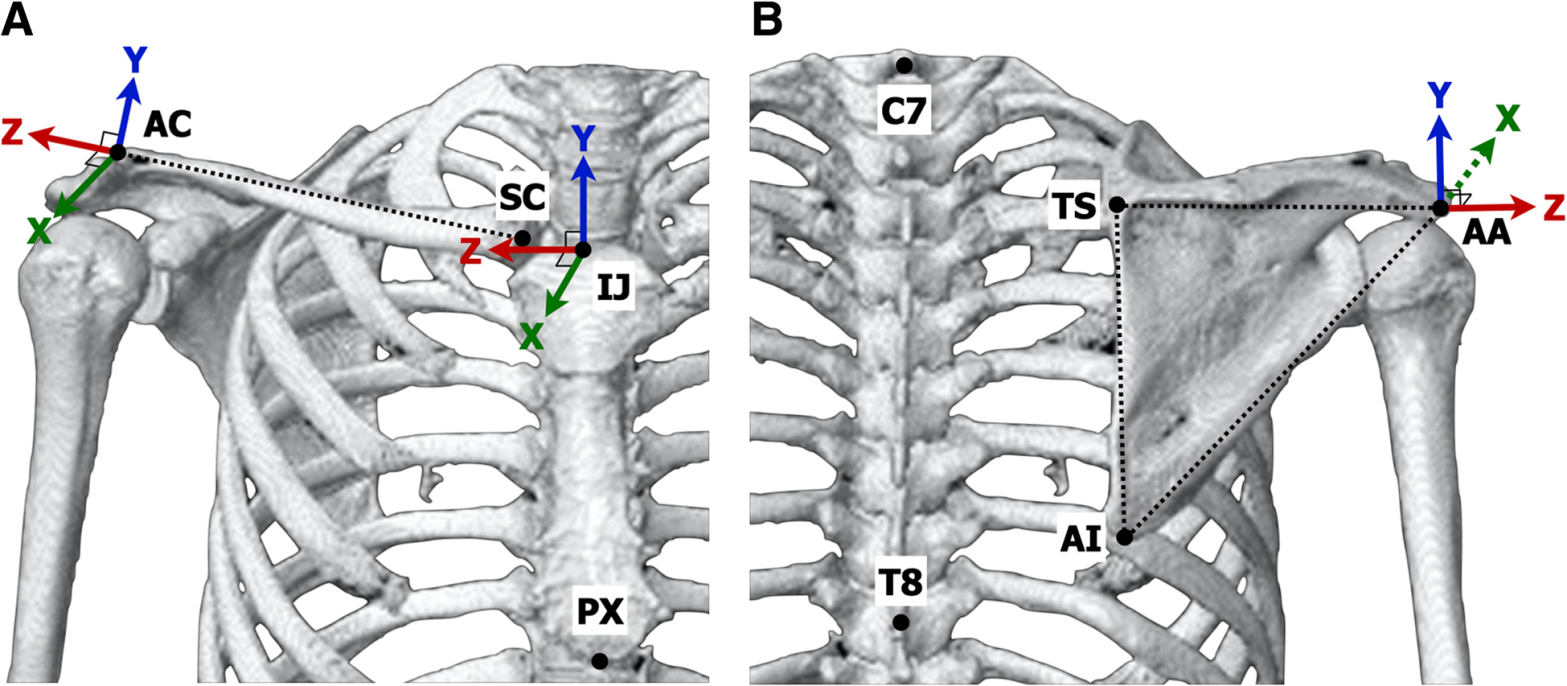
The local 3-dimensional coordinate systems of the thorax, clavicle, and scapula. a The thorax and clavicular coordinate systems are defined from the specific bony landmarks on CT scans, and the angular rotation of the clavicle relative to the thorax is calculated. IJ, sternal notch; PX, xiphoid process; SC, sternoclavicular joint; AC, acromioclavicular joint. b The scapular coordinate systems are defined from the specific bony landmarks of the scapula, and the angular rotation of the scapula relative to the thorax is calculated. C7, 7th cervical vertebra; T8, 8th thoracic vertebra; TS, root of the scapular spine; AI, inferior angle of the scapula; AA, posterolateral edge of the acromion
